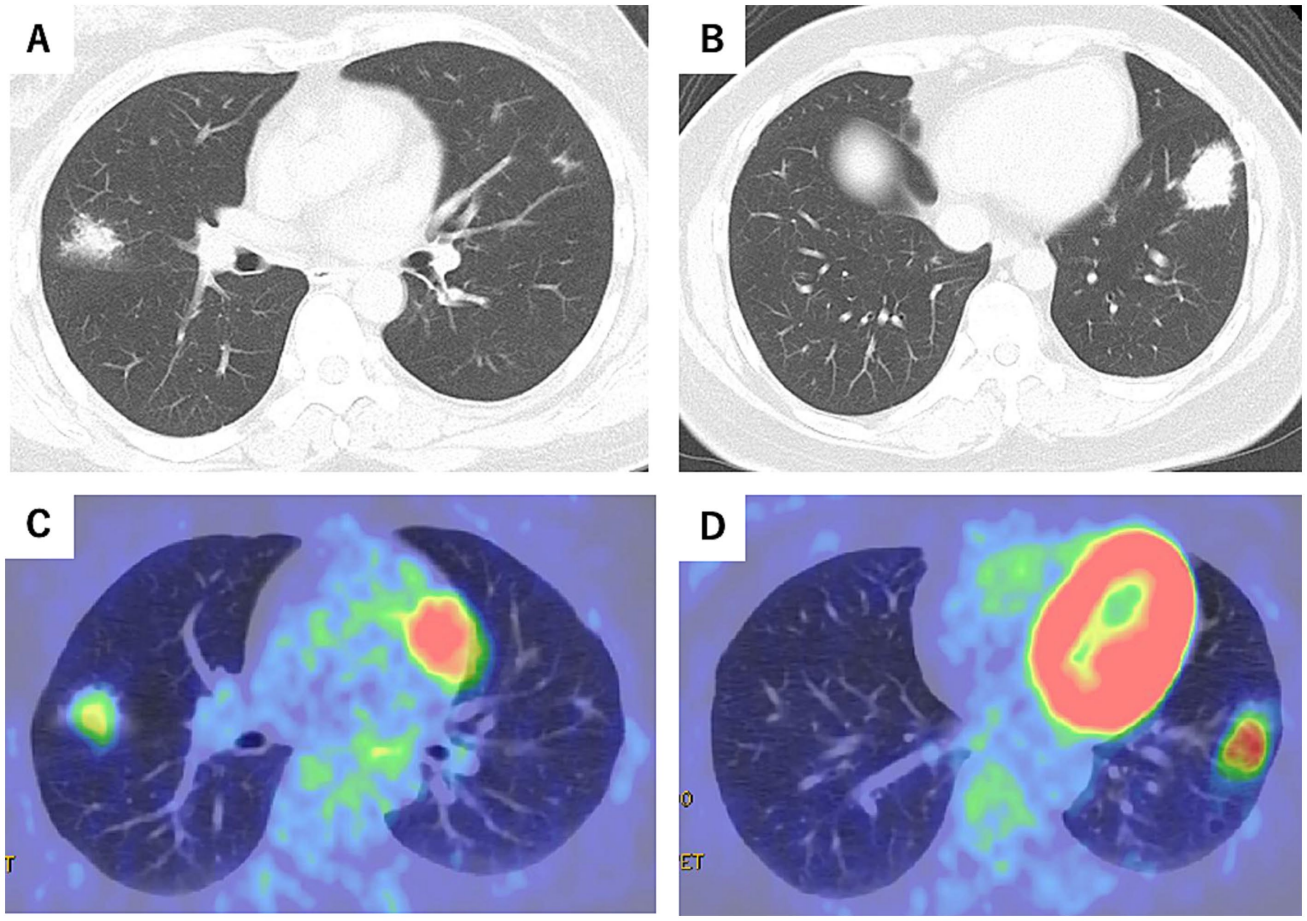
Chest CT at the initial visit (Day 0) (A,B) and positron emission tomography-computed tomography (PET-CT) scans (C,D) (October X-year). Irregular nodular lesions were observed in the right middle lobe (S4), measuring 20 mm, with a maximum standardized uptake value (SUVmax) of 2.78 on PET-CT. Similar nodular lesions in the left lower lobe (S8), measuring 35 mm, showed an SUVmax of 4.53.
